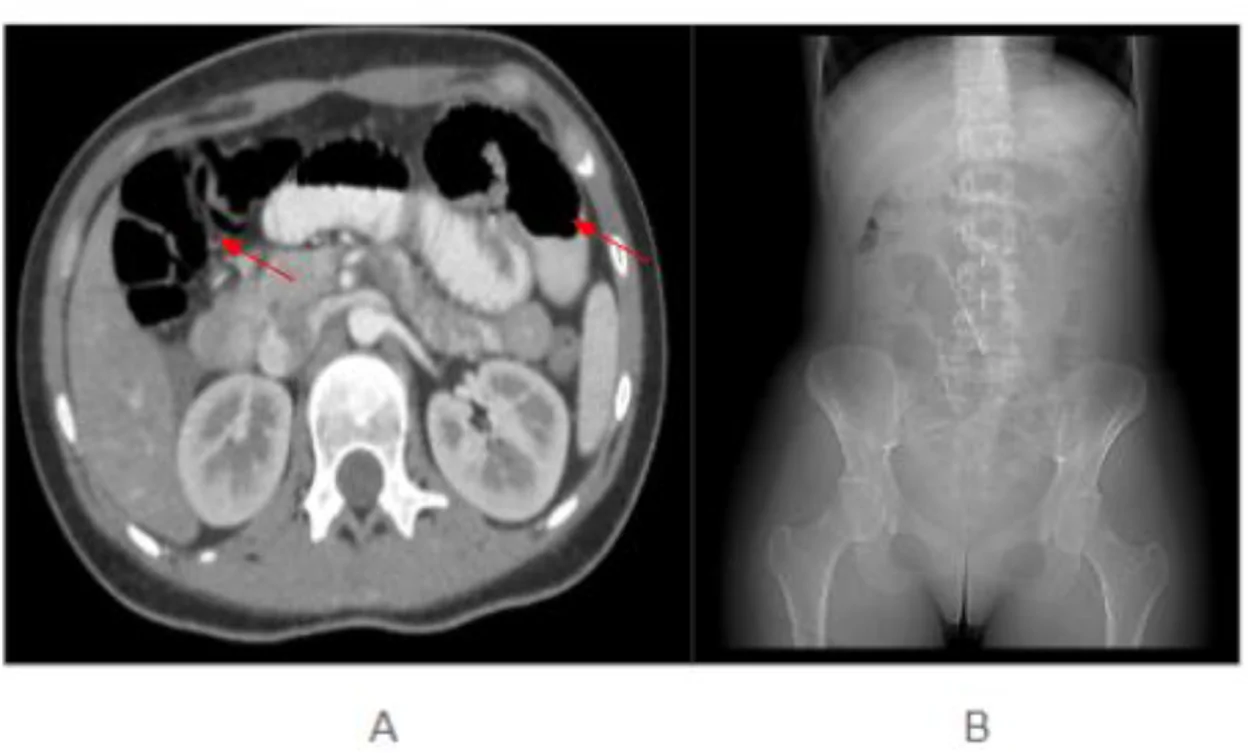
Computed tomography scan of the abdomen with intravenous contrast. (A) Transverse reconstruction. Arrows showing dilated intestinal loops. (B) Coronal reconstruction. Source: photographic images taken by the authors.
